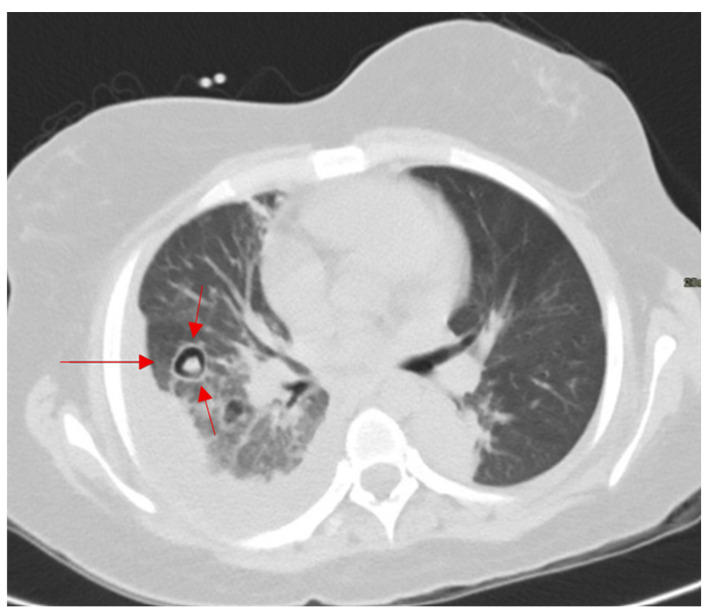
Axial CT of the chest showing a nodular opacity with retracted infarcted lung and crescentic and circular cavitation in pulmonary aspergillosis (red arrows).
